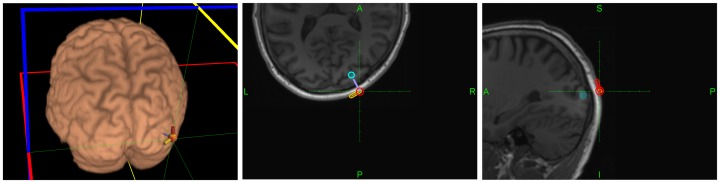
Location and orientation of hot spot for phospene production overlaid on MRI image of the head of subject 2 (see Figure 2). The active direction producing phospenes is highlighted in orange (in red, the orthogonal direction not producing phosphenes).
